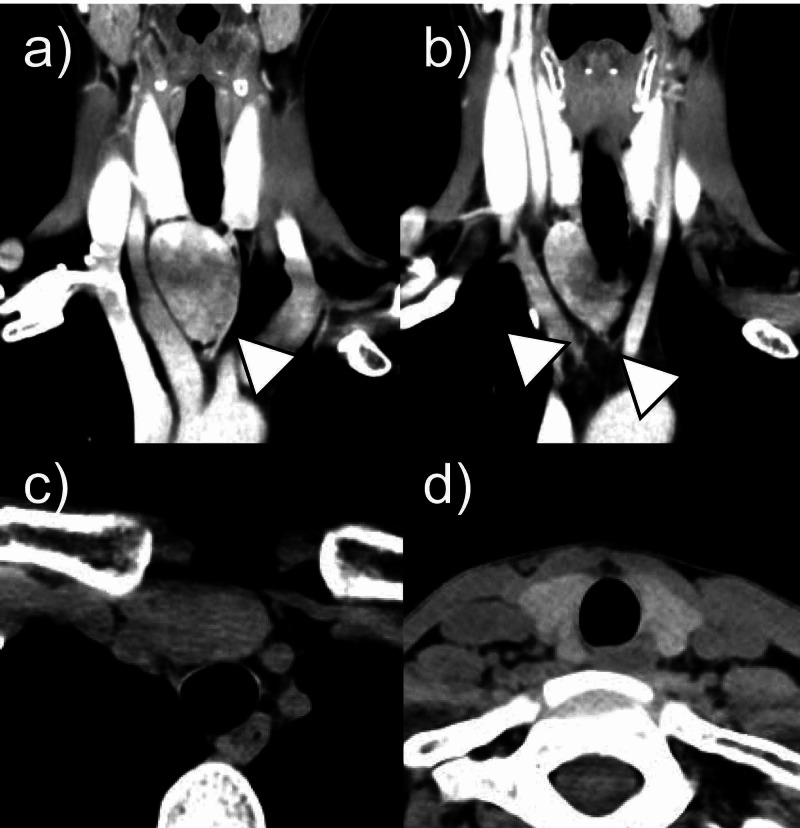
CT findings of the present case. (a, b) Contrast-enhanced CT coronal image. The arrowheads indicate the vasculatures entering the mass from the thoracic sides. (c) Plain CT findings of the mass located in the mediastinum. (d) Plain CT findings of the orthotopic thyroid gland.
